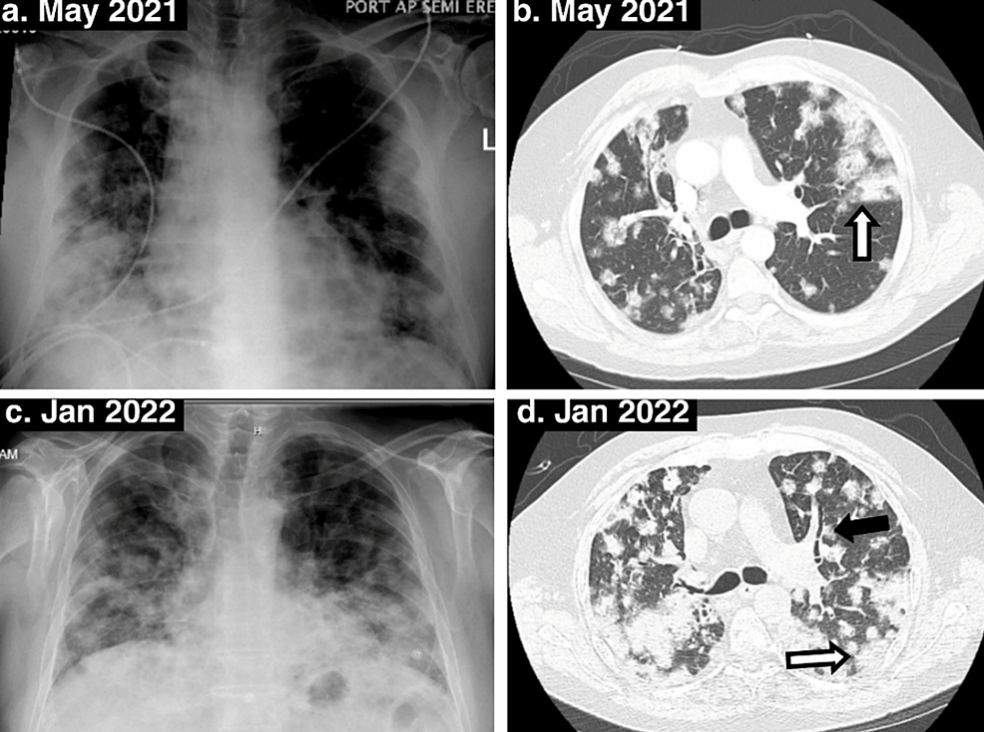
Initial and subsequent radiographic images demonstrating the progression of multiple, bilateral, irregular nodular densities with a predominantly peripheral distribution (white arrows) and air-bronchograms (black arrow), no pathologically enlarged hilar, mediastinal, or axillary lymphadenopathy.
